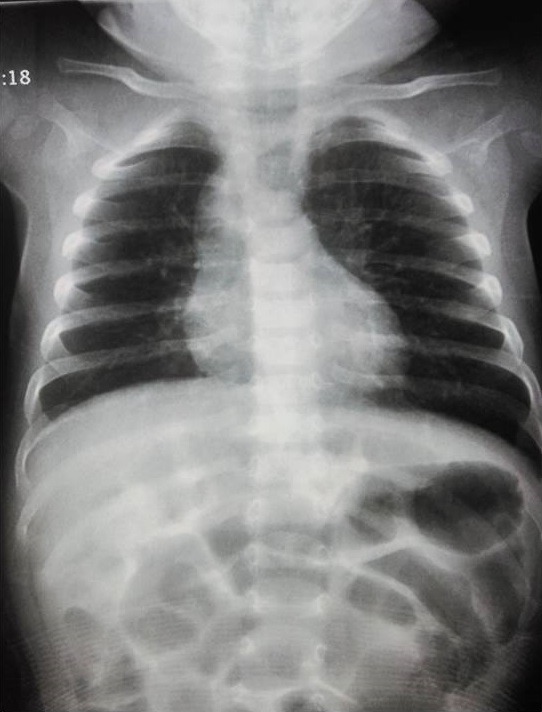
Radiographie du thorax montrant une distension thoracique avec effacement du bouton aortique
